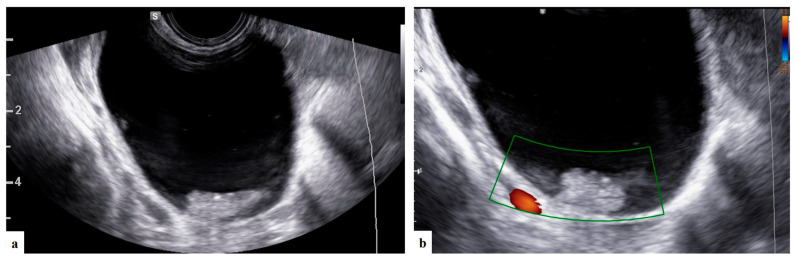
Grayscale and color Doppler ultrasound (a,b) images showing unilocular solid mass with anechoic content and papillary projection suspected of serous cystoadenofibroma (patient n° 5).
